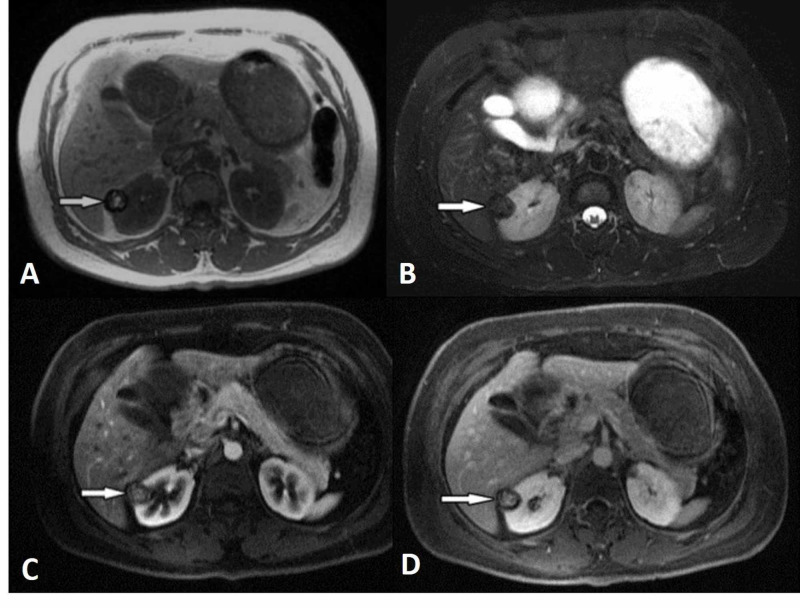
Papillary renal cell carcinoma (arrows) Unenhanced axial T1-weighted (a), fat-saturated T2-weighted (b), and contrast-enhanced T1-weighted (c, d) magnetic resonance images show a well-demarcated, hypovascular cortical lesion with a hemosiderin rim in T1-weighted images in the right kidney.
